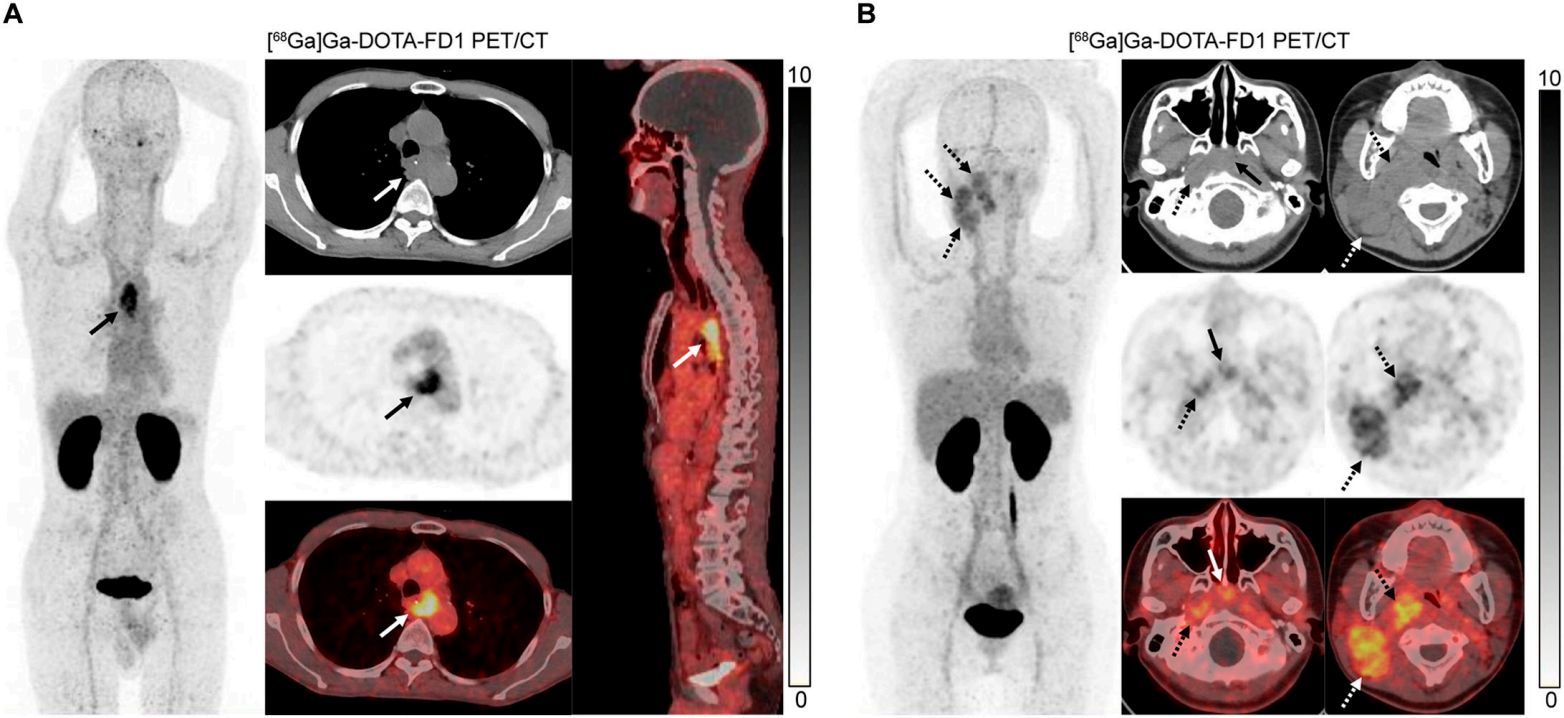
(A) An adult patient with known esophageal cancer underwent [^68^Ga]Ga-DOTA-FD1 PET/CT for initial assessment. Intense [^68^Ga]Ga-DOTA-FD1 uptake was observed in the primary lesion (black and white arrows). (B) An adult woman with known nasopharyngeal carcinoma who underwent [^68^Ga]Ga-DOTA-FD1 PET/CT for initial assessment. Intense [^68^Ga]Ga-DOTA-FD1 uptake was observed in the primary lesion (black and white arrows) and cervical lymph node metastases (black and white dotted arrows).
